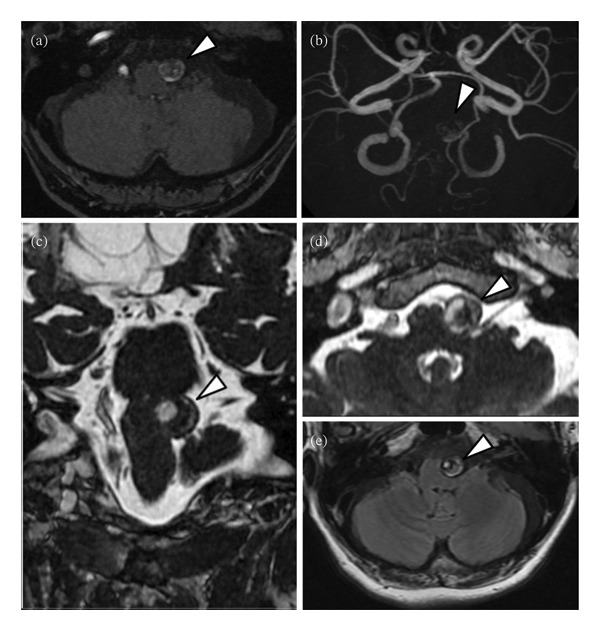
Preoperative magnetic resonance imaging (MRI). Magnetic resonance angiography (MRA) time‐of‐flight image (axial (a); maximum intensity projection (b) shows a large left vertebral artery partially thrombosed aneurysm (13.2 × 13.5 mm). Gadolinium (Gd)−enhanced fast imaging employing steady state acquisition (FIESTA) (coronal (c); axial (d)) shows the aneurysm compressing the brain stem from left to right. Fluid attenuated inversion recovery (FLAIR) (e) shows no brain stem edema due to the mass effect of the aneurysm. Arrowhead: partially thrombosed aneurysm.
